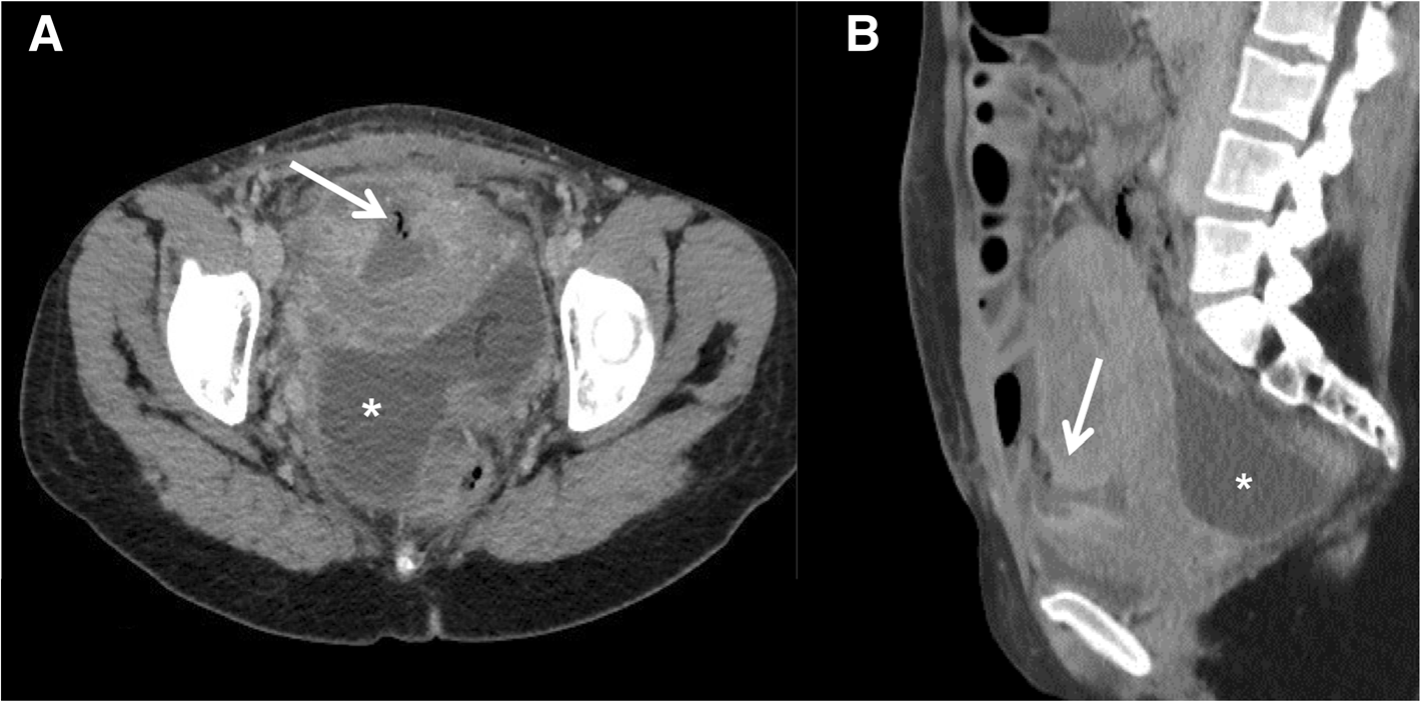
Uterine rupture: CT examination (a, b) showed the presence of gas within the uterine defect, extending from the endometrial cavity to the extra uterine parametrium (white arrow), in association with hemoperitoneum (asterisks). In the appropriate clinical setting, these features are highly suspicious for uterine rupture
